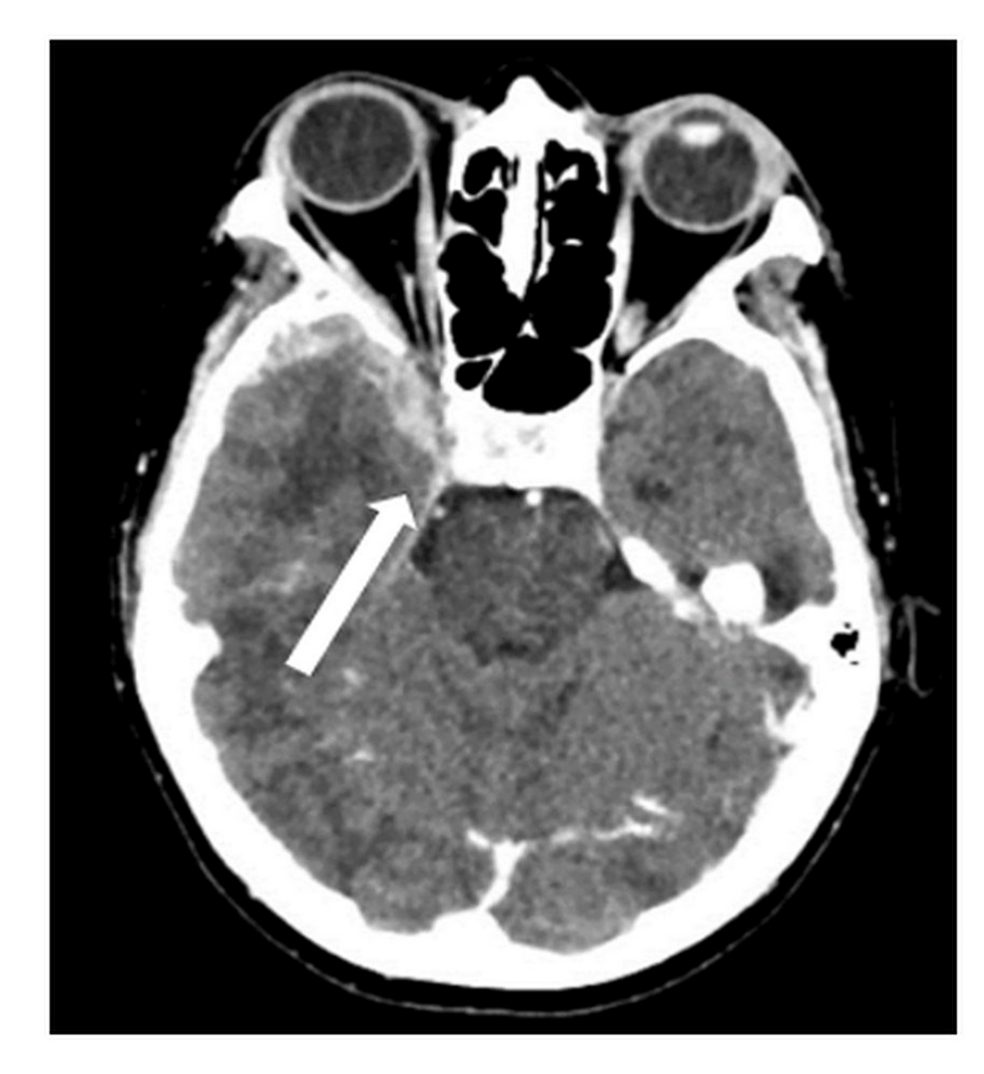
A contrast-enhanced CT scan and orbit (axial view) showed the presence of a lesion seen along the dura of the right frontal and right temporal regions, approaching the right orbital apex.
